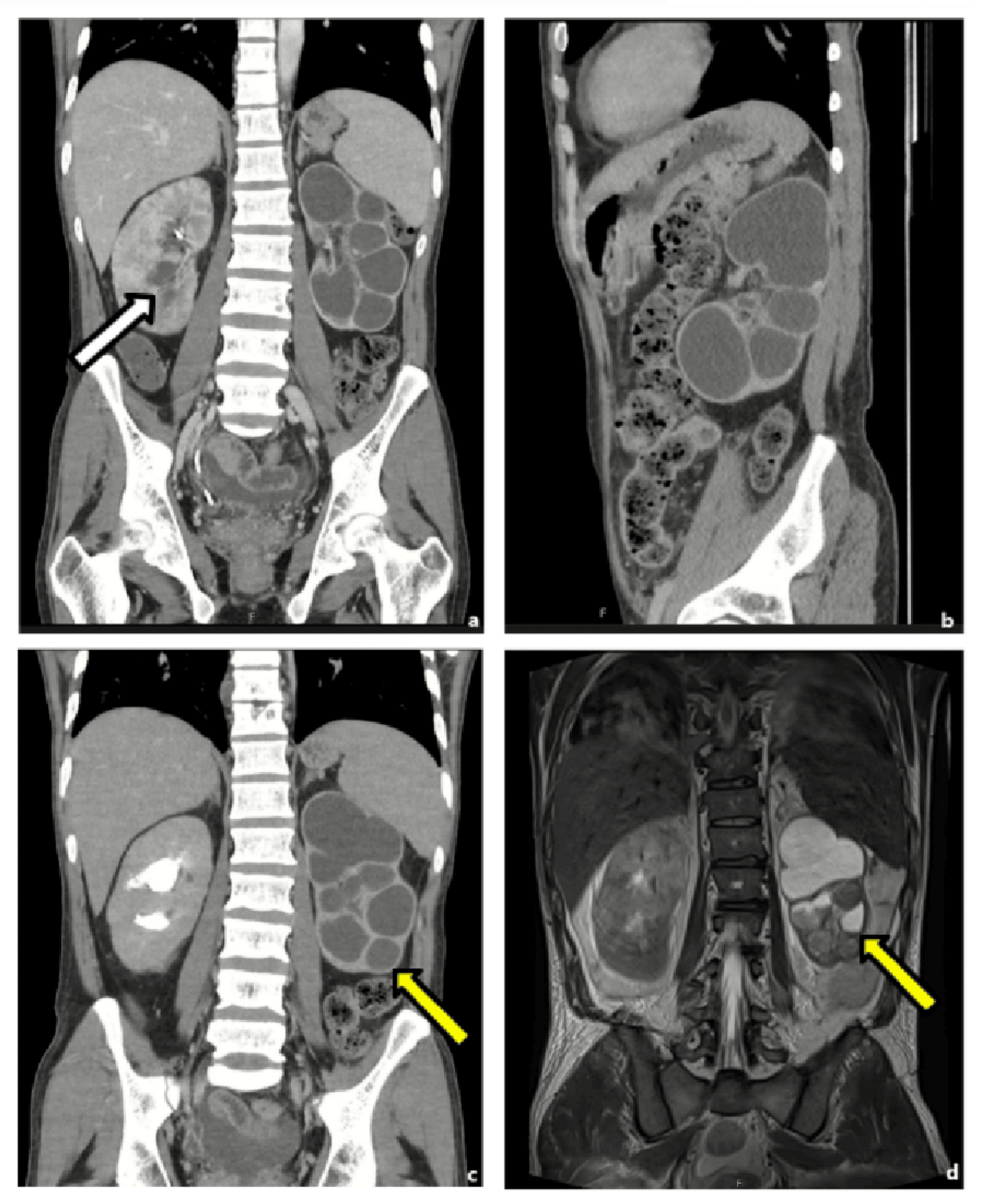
CT urogram in the coronal (a) and sagittal (b) plane after contrast admin in the portal phase and in the coronal plane in the excretory phase (c), and T20weighted MRI on the coronal plane (d). The left kidney exhibited minimal residual parenchyma extensively replaced by apparent caliectasis (yellow arrow), while the right kidney displayed ureteral pyelitis (white arrow).
